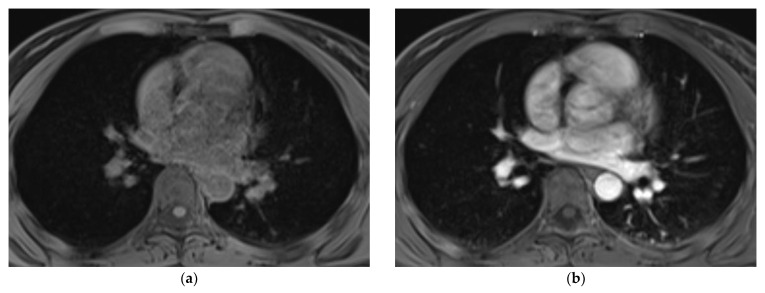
Example of the assessment of the lung in the 20-min-WB-MRI-protocol: example of axial pre- (a) and post-contrast T1w VIBE Dixon of the thorax (b) in a 25-year old patient with malignant melanoma and currently no evidence of disease. Please note that the images have been cropped, as the skin tissue was part of the acquisition.
